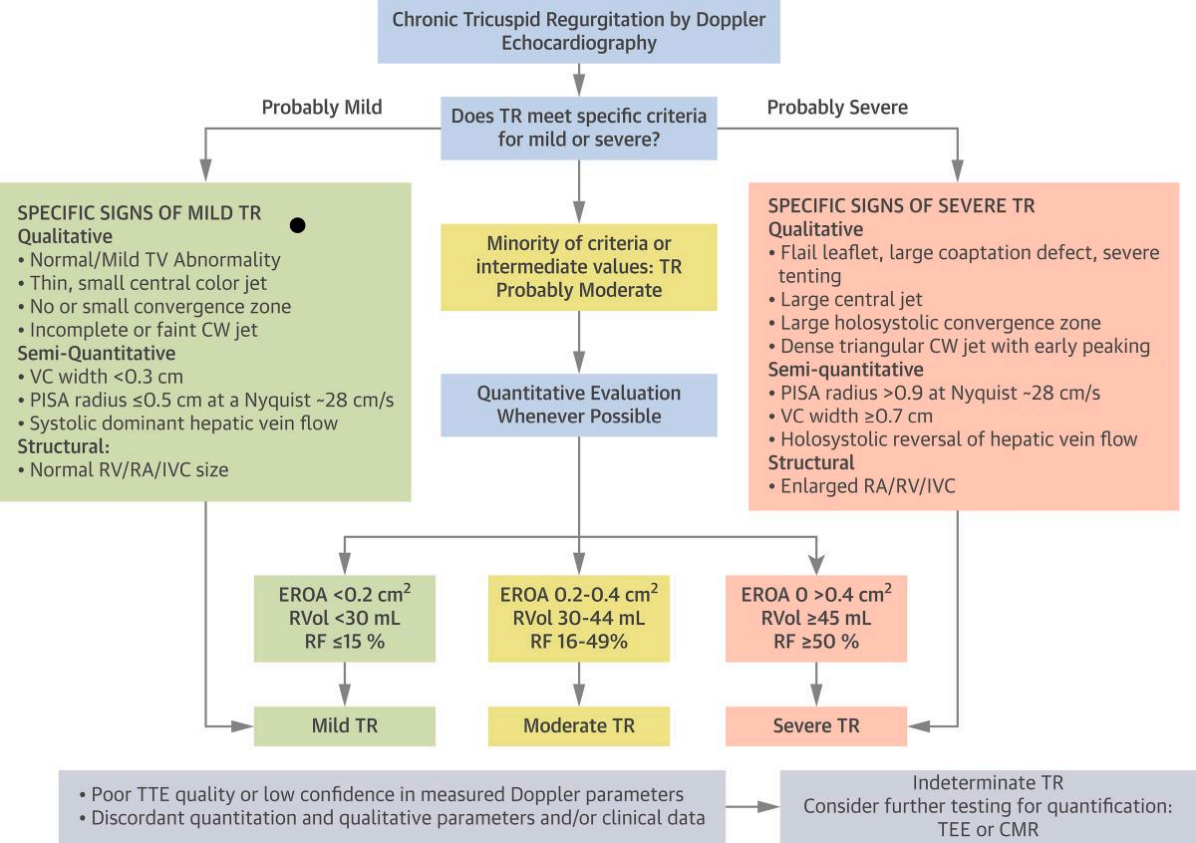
Algorithm for the integration of multiple echocardiographic parameters of TR severity (reprinted from^15^, with permission from Elsevier). CW, continuous wave; EROA, effective regurgitant orifice area; IVC, inferior vena cava; PISA, proximal isovelocity surface area; RA, right atrium; RV, right ventricle; RVOL, regurgitation volume; TR, tricuspid regurgitation; TV, tricuspid valve; VC, vena contracta.
